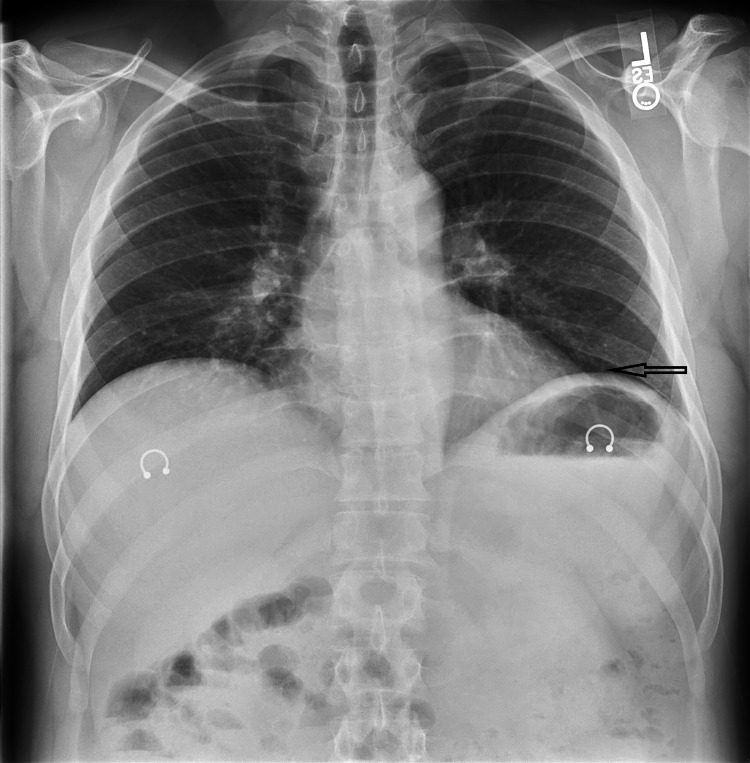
Chest X-ray showing faint infiltrate on the left lung base (arrow)
